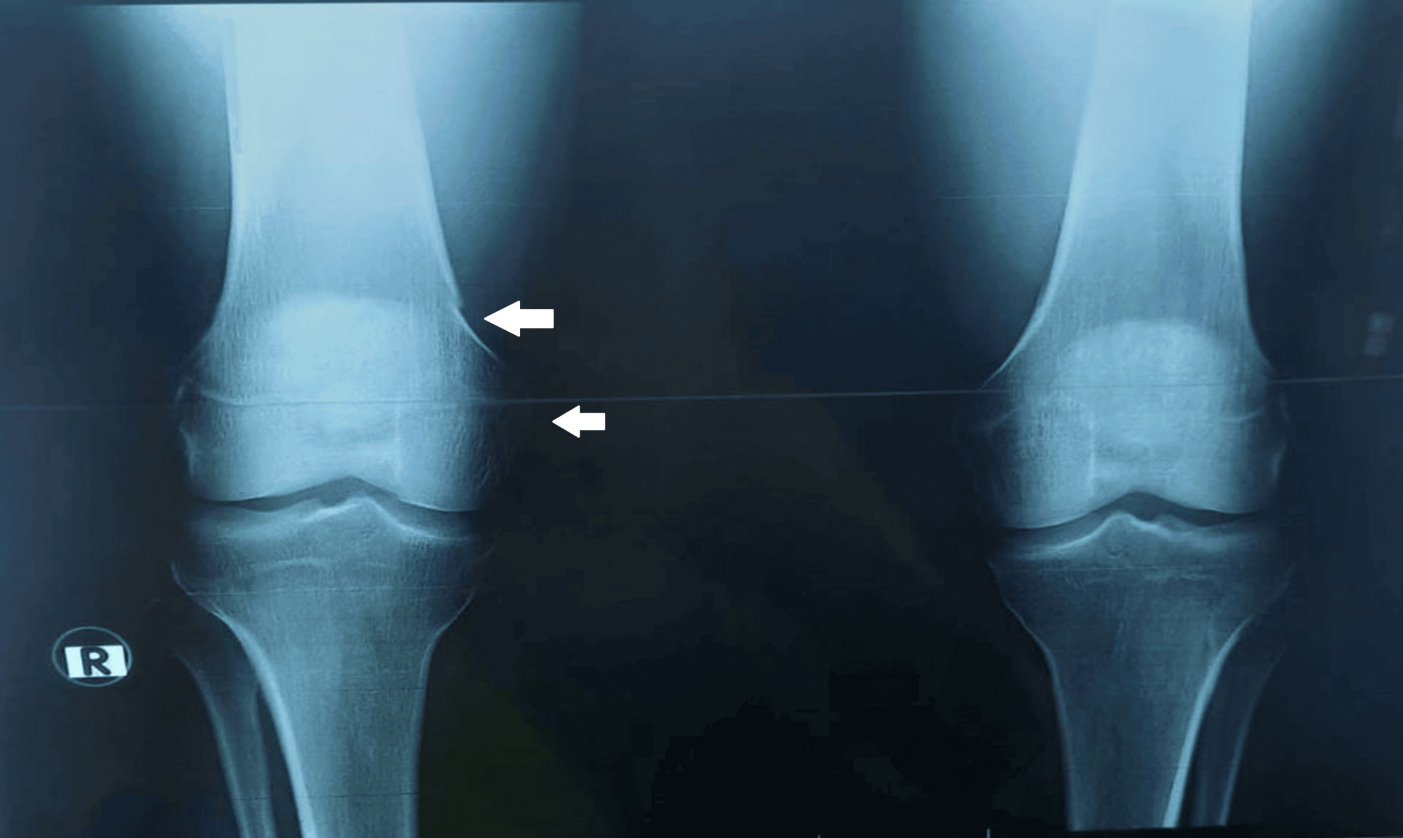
X-ray of the right knee showing osteolysis of the medial condyle.
